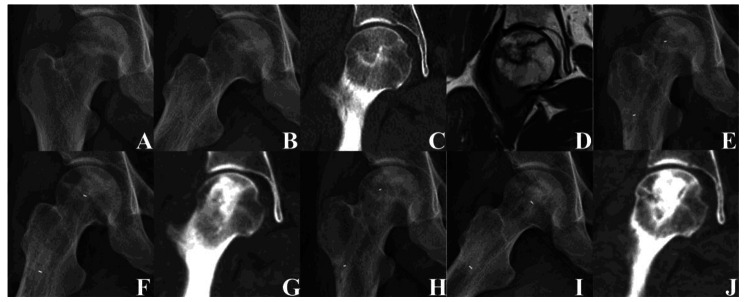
Images of a 30-year-old man with type 3 right steroid-induced osteonecrosis of the femoral head. (A–D) Preoperative X-ray and computed tomography showed extensive necrosis of the femoral head with mild collapse. (E–G) Three days after the operation, radiographs showed that the femoral head was still round. (H–J) Two year after the operation, radiographs and computed tomography showed that the femoral head was round and the bone density had increased
